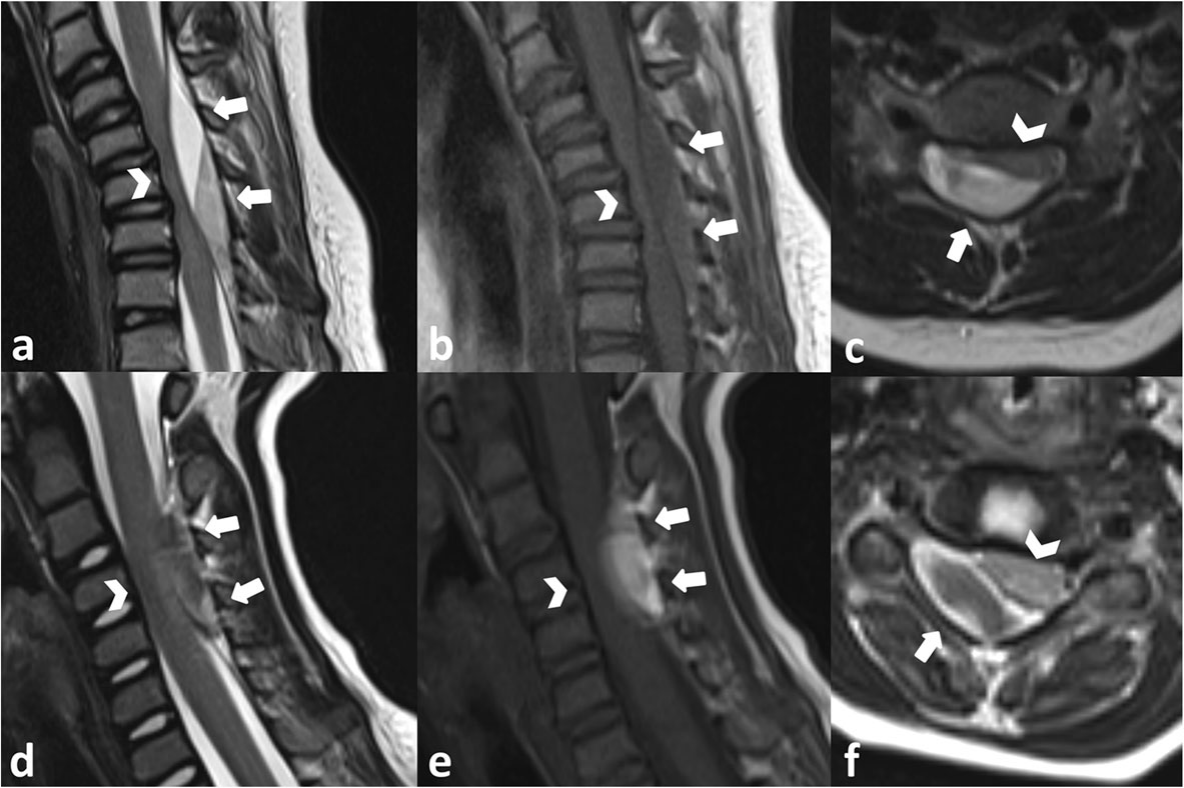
MRI scans of case 1 and case 2. In case 1 (a–c) a hyperacute epidural hemorrhage was revealed (T1w isointense signal (1b) and T2w slightly hyperintense signal (1a)). In case 2 (d–f) a subacute hemorrhage was found (T1w hyperintense (2b), and T2w iso- to slightly hyperintense signal (2a)). Arrows: epidural hemorrhage, arrowheads: spinal cord.
